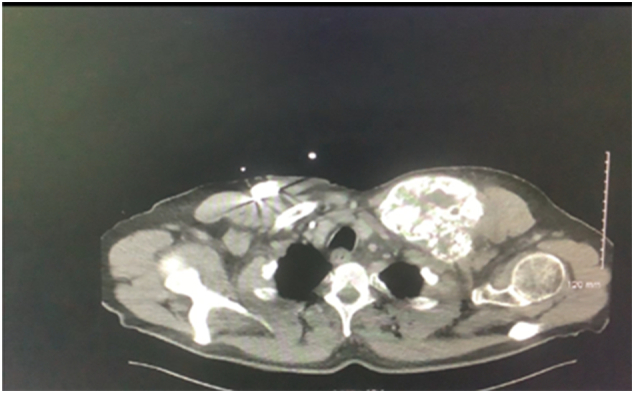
Contrast-enhanced CT-scan for left clavicular 10 × 8 cm.
